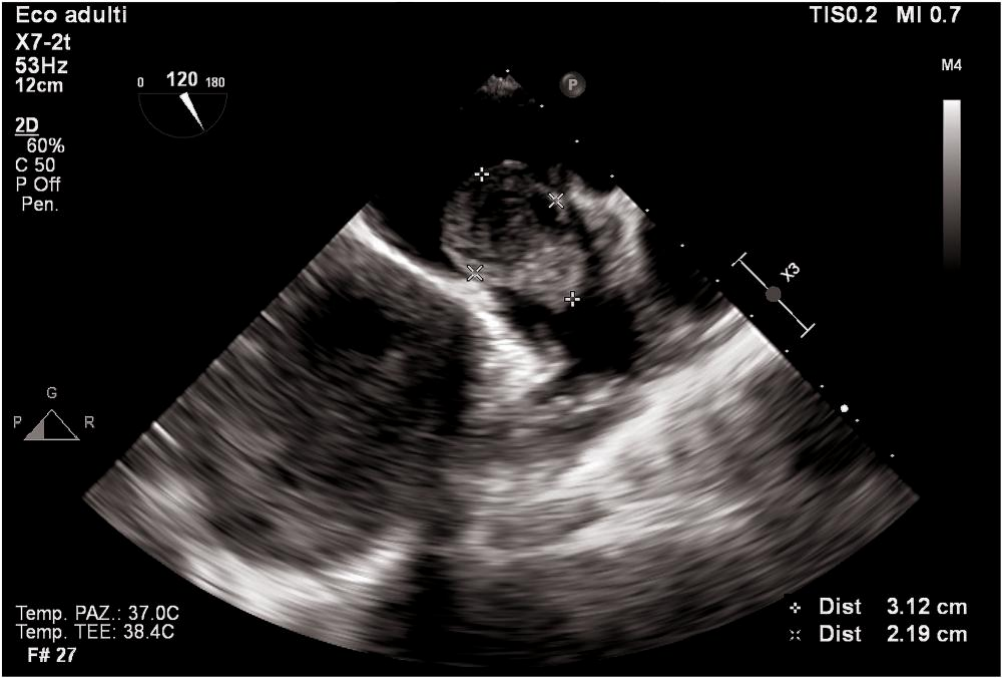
Transoesophageal echocardiogram mid-oesophageal view at 120°. The figure shows a huge mass in the left atrial appendage.
